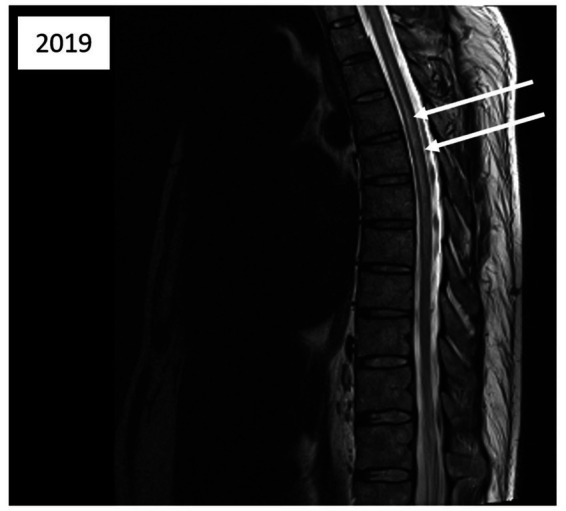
Magnetic resonance imaging of the thoracic spinal cord; sagittal T2-weighted images acquired 5 days after the second diving incident reveal abnormalities in spinal cord signal intensity (indicated by arrows).
